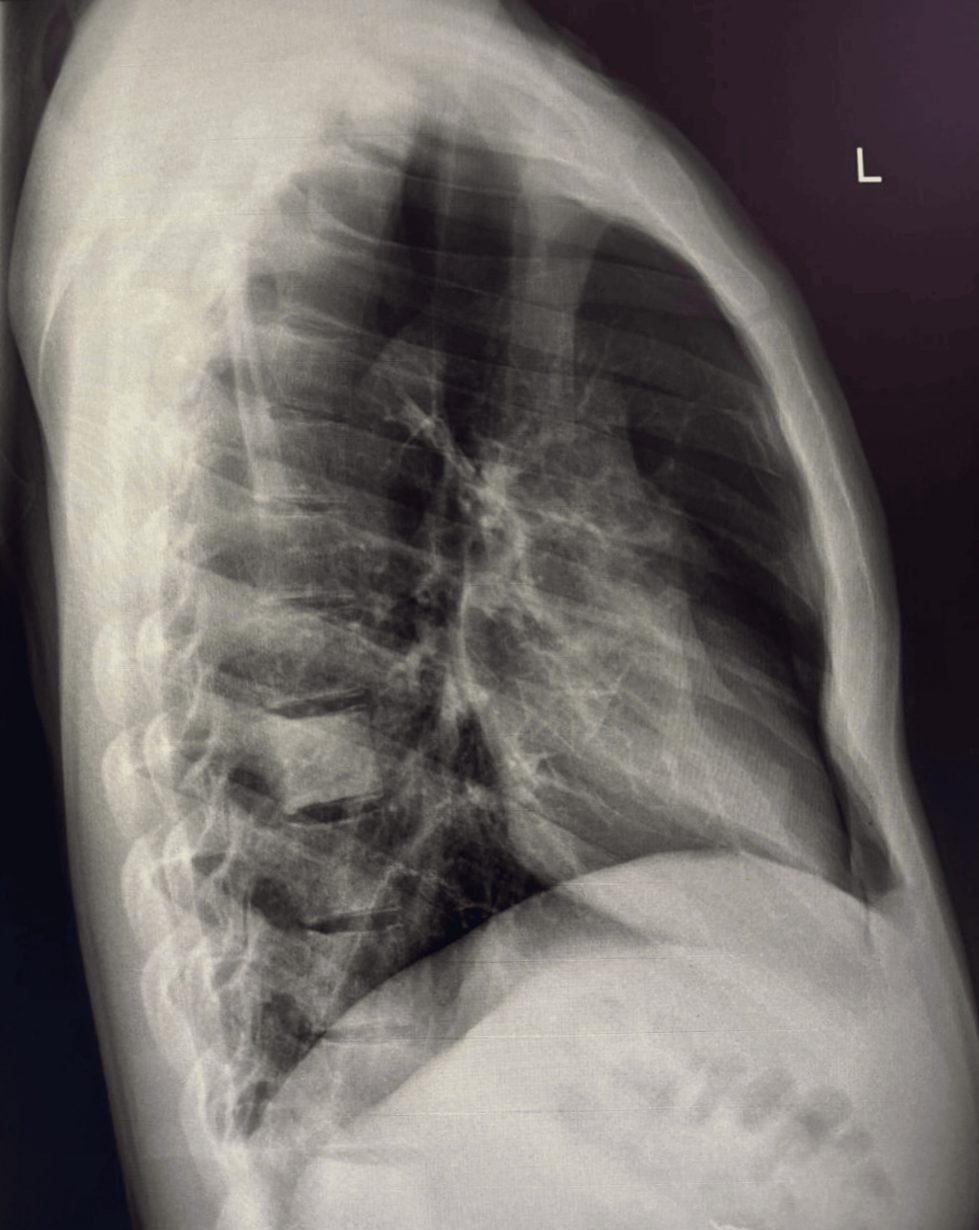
Chest X-ray lateral view.
